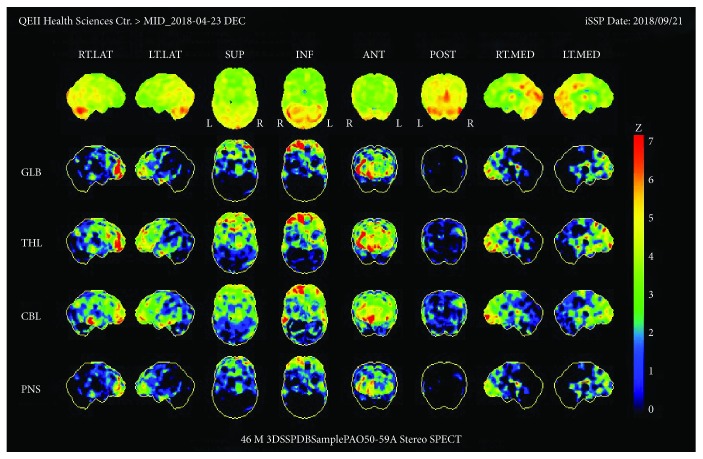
Colour scale brain perfusion SPECT analysis with 99mTx-HMPAO for patient 3.
